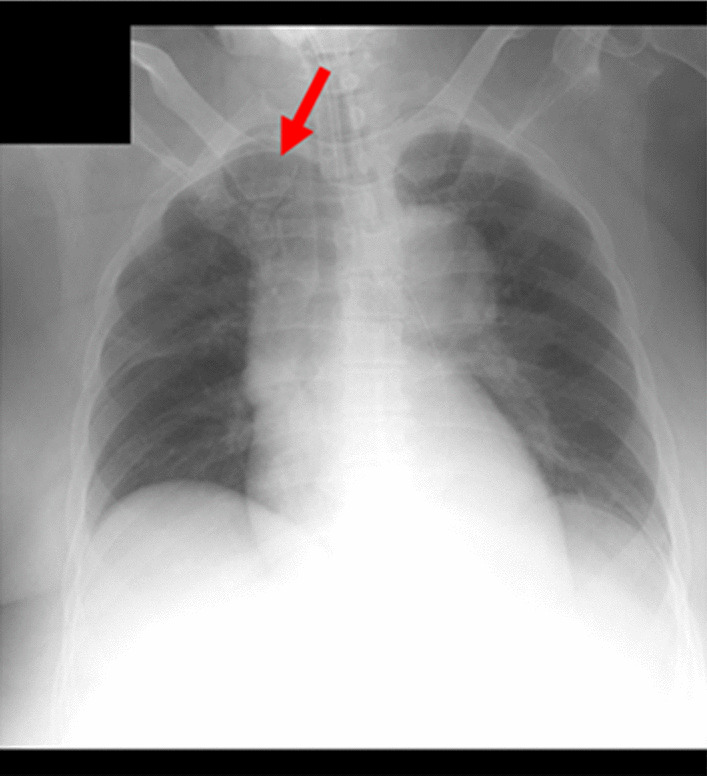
Chest radiograph after pulmonary rehabilitation. Red arrow indicates amelioration of atelectasis
